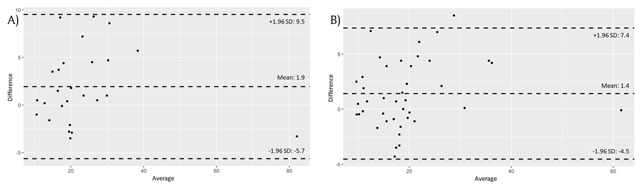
(A) Bland-Altman plots of prostate volume as measured by the two readers on baseline CT scans. (B) Bland-Altman plots of prostate volume as measured by two readers on the follow-up CT scans.
